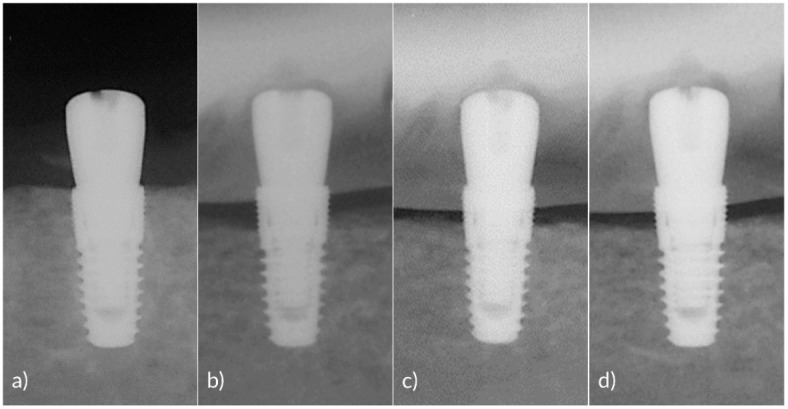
Peri-apical radiographs taken at (a) implantation (Surgery), (b) after inducing peri-implantitis (Baseline, T1), (c) 4 weeks after the first administration (T2), and (d) 4 weeks after the second administration (T3).
